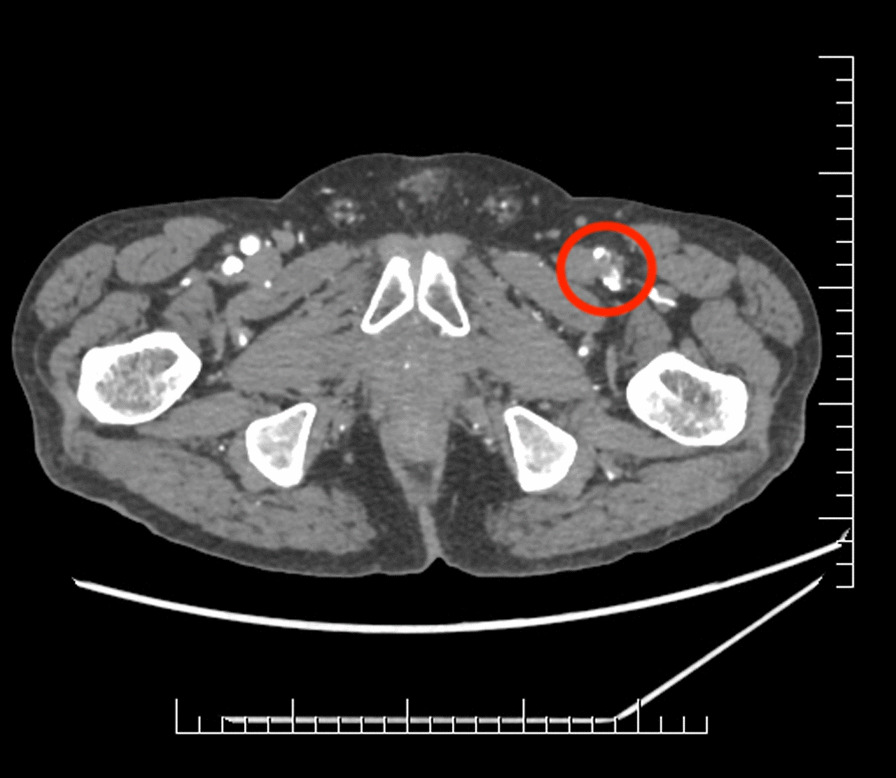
CT angiography showing a saddle-shaped thrombus (in the red circle) in the bifurcation of the left femoral communis artery
